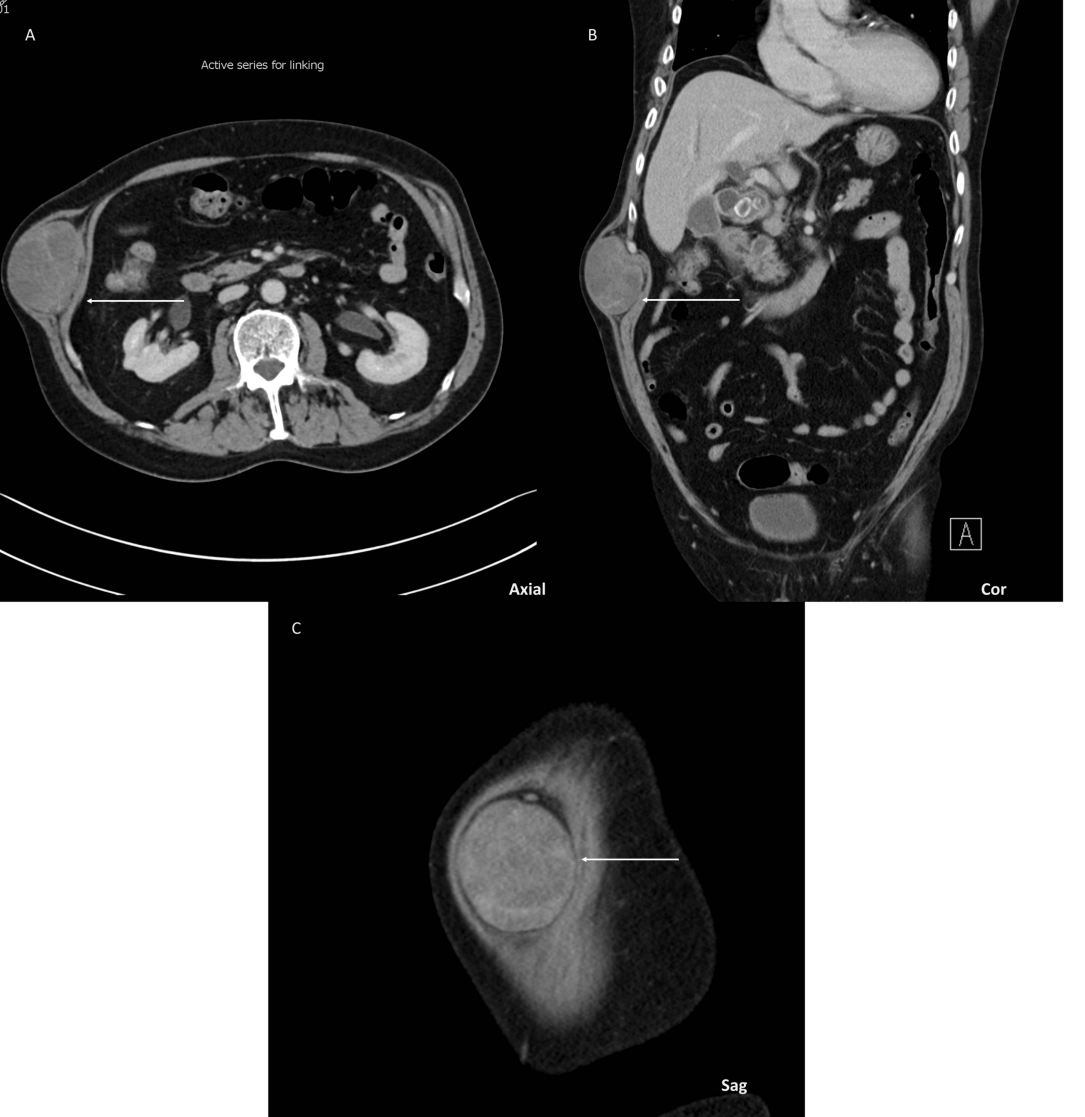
Abdominal/pelvic CT imaging of the abdominal wall mass. (A-C) Axial, coronal, and sagittal images of subsequent CT with soft tissue window showing a subcutaneous soft tissue mass along the right thoracoabdominal wall below the level of the right 12th rib. The mass demonstrated mixed density (arrows).
